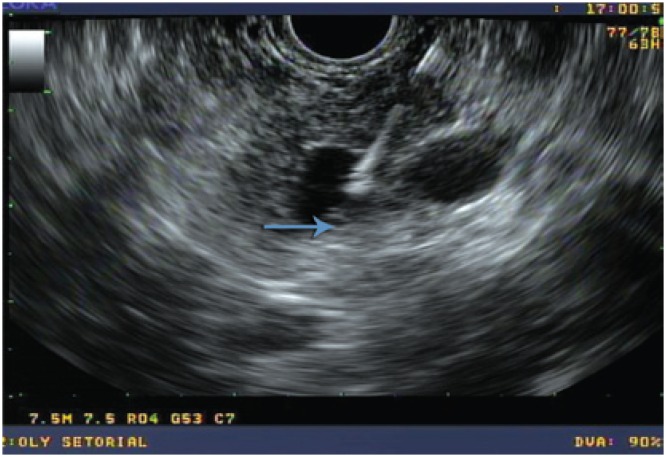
Endoscopic ultrasound-guided fine needle aspiration of the cyst wall
